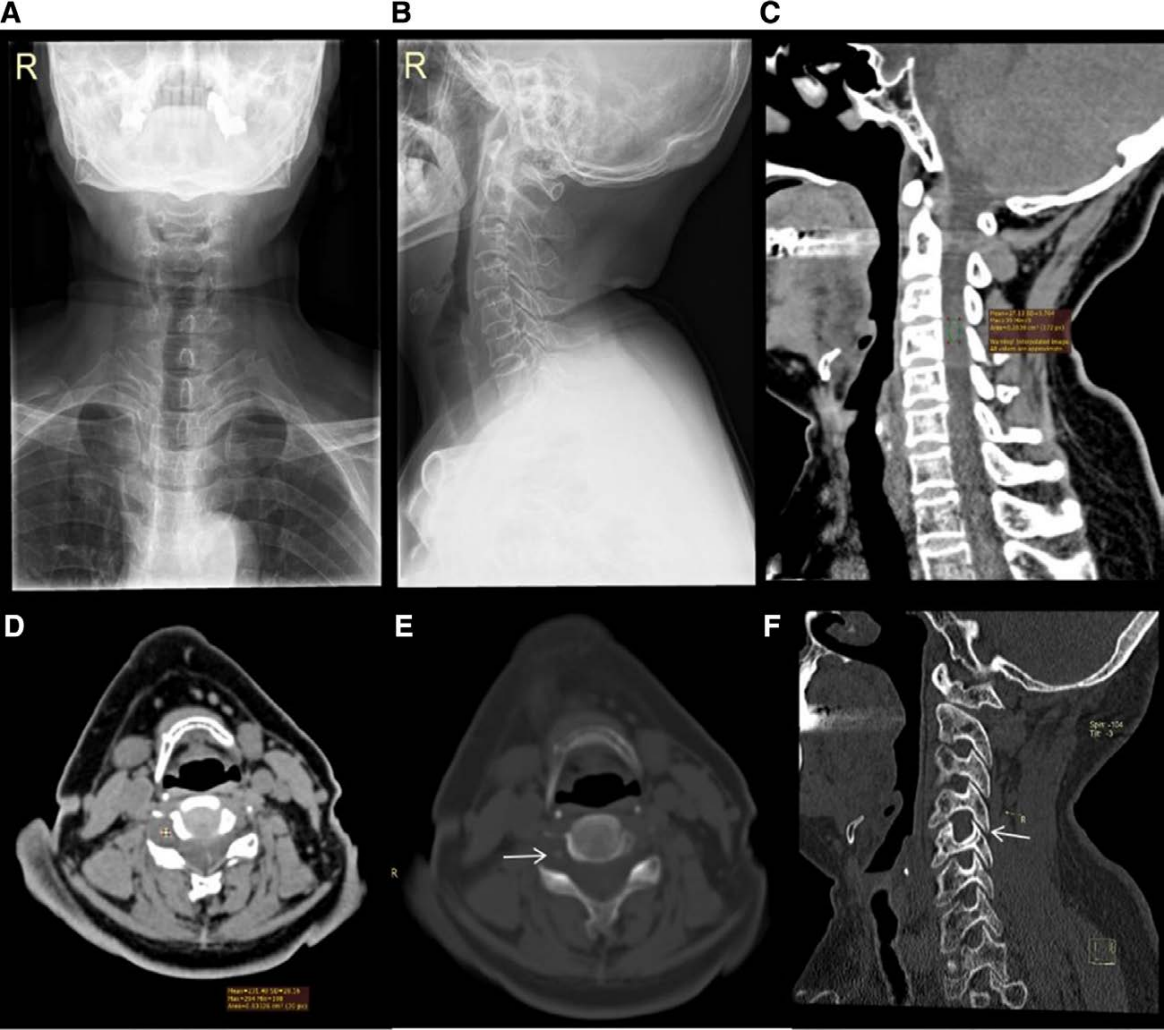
X-ray and CT of the cervical spine in a 60-yr-old female. Views: (A) X-rays in the posteroanterior and (B) lateral positions showing no obvious abnormalities. (C) CT sagittal soft tissue window showing that most of the tumor has a soft tissue density, with a CT value of approximately 27.13 HU. (D) CT axial soft tissue window showing irregular calcification in the tumor, with a CT value of approximately 231.40 HU. (E) CT axial bone window showing calcification (white arrow) in the tumor. (F) CT oblique sagittal bone window showing an enlarged intervertebral foramen at cervical 4/5 (white arrow). The adjacent bone is compressed and thin. CT = computed tomography.
